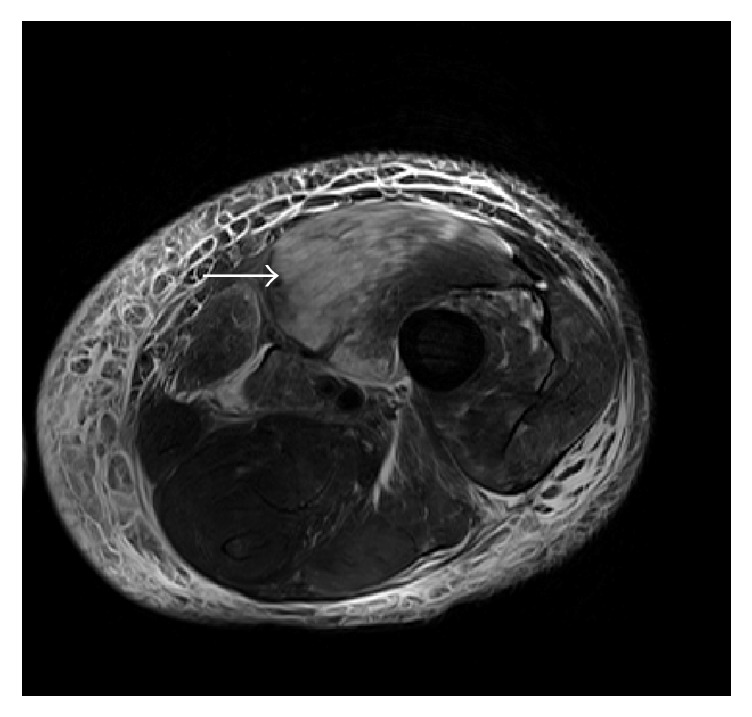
MRI of the left thigh, coronal section, showing hyperintense signals on T2 weighted images (white arrow).
